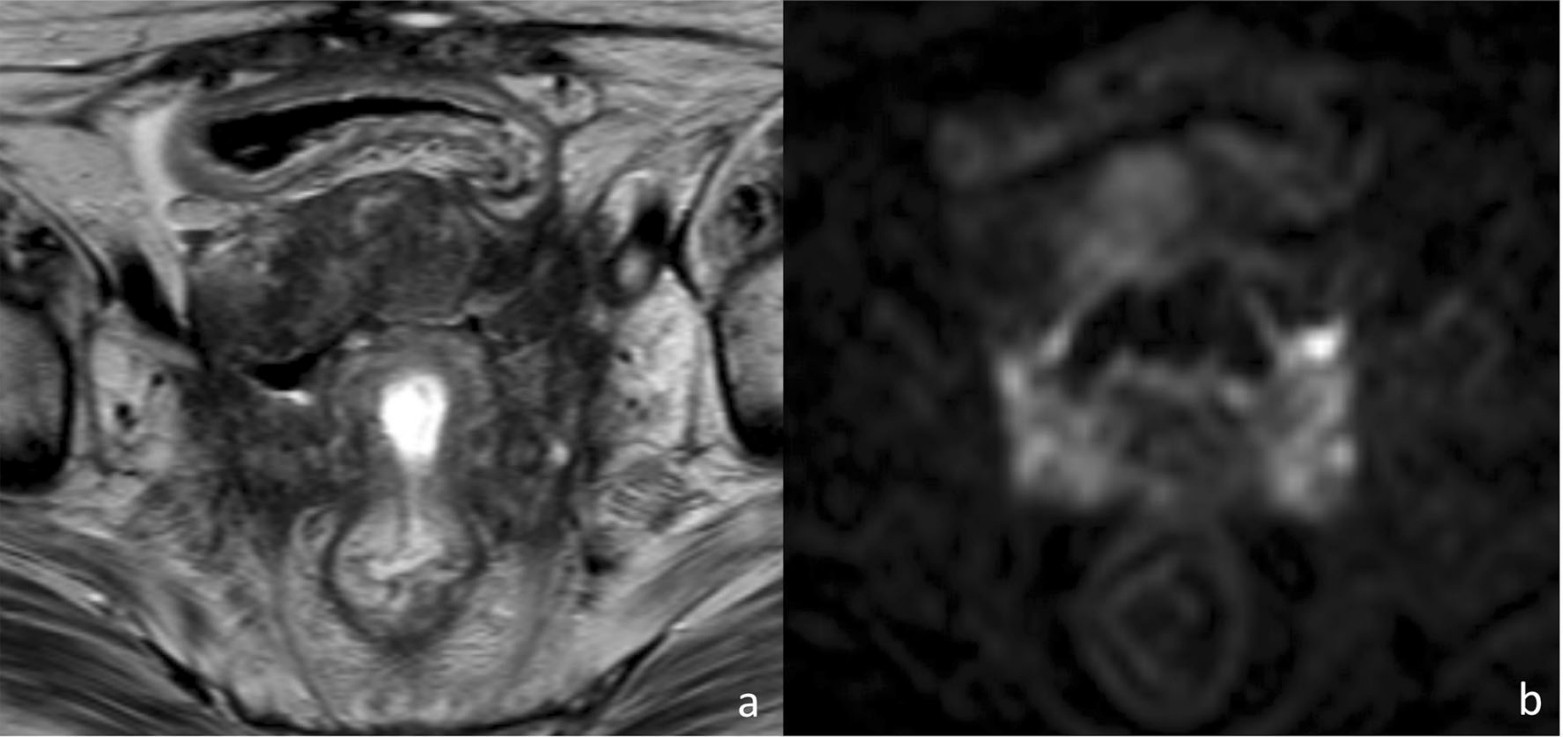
Fifty-two-year-old female patient with stage IVa cervical cancer after chemoradiation therapy, depicted in the axial plane, both on high-resolution T2-weighted (a) and diffusion-weighted (b) images. A clear preferential spread within the bow-tie-shaped Mullerian compartment, with extensive involvement of the mesometria, is seen
